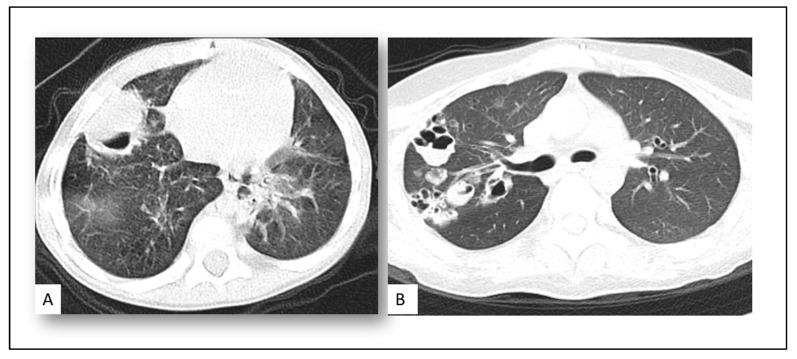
Acute myeloid leukemia patient with COVID-19-associated pulmonary aspergillosis with CT chest showed cavitary lung lesion and serum galactomannan > 1 (A). Patient with brain tumor with COVID-19-associated pulmonary aspergillosis with CT chest showed multiple cavitary lung lesions and BAL galactomannan > 1 (B).
